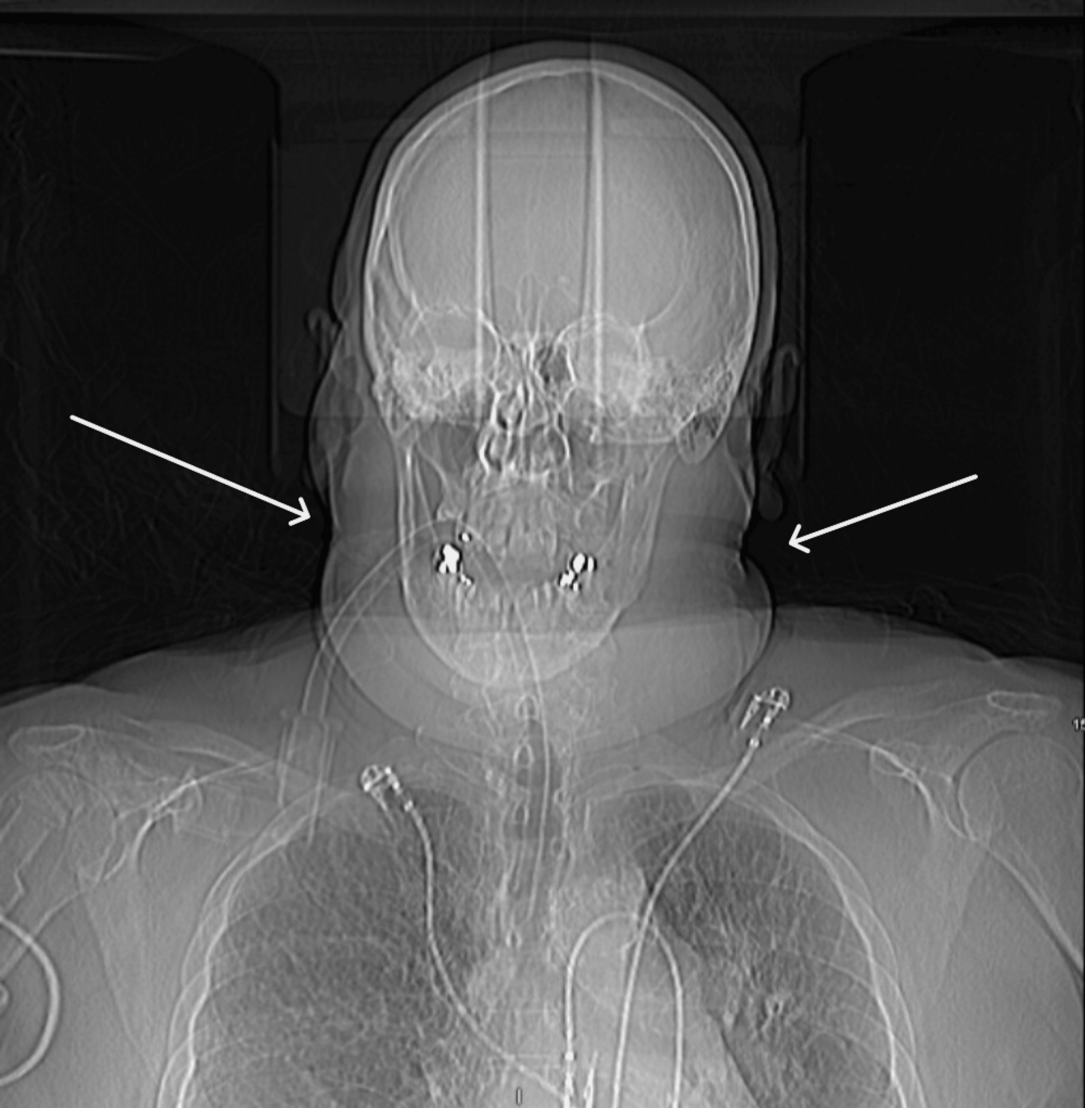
Computed tomography scan image showing significant neck swelling (arrows)
